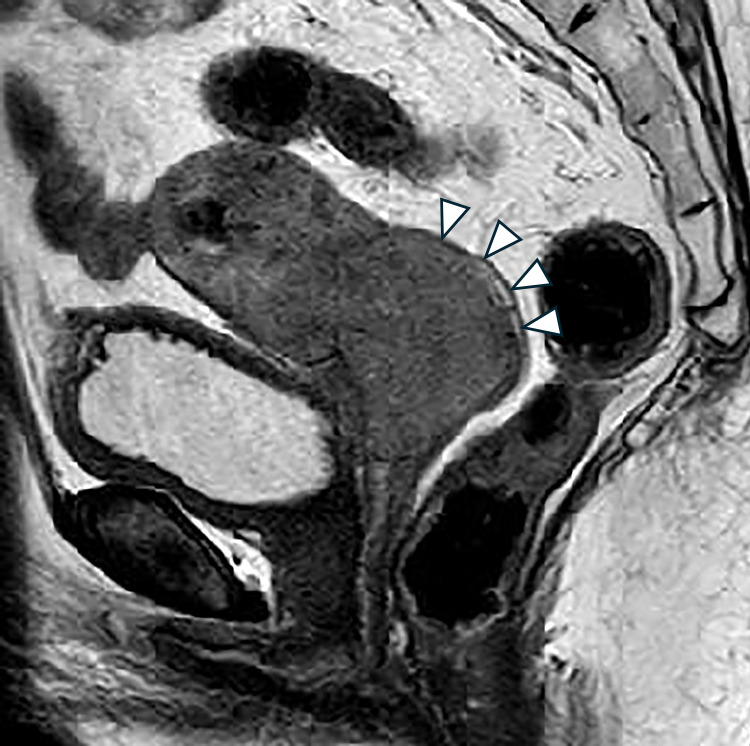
Non-contrast sagittal T2-weighted MRI of the pelvic region showing a cervical mass (arrowhead).
